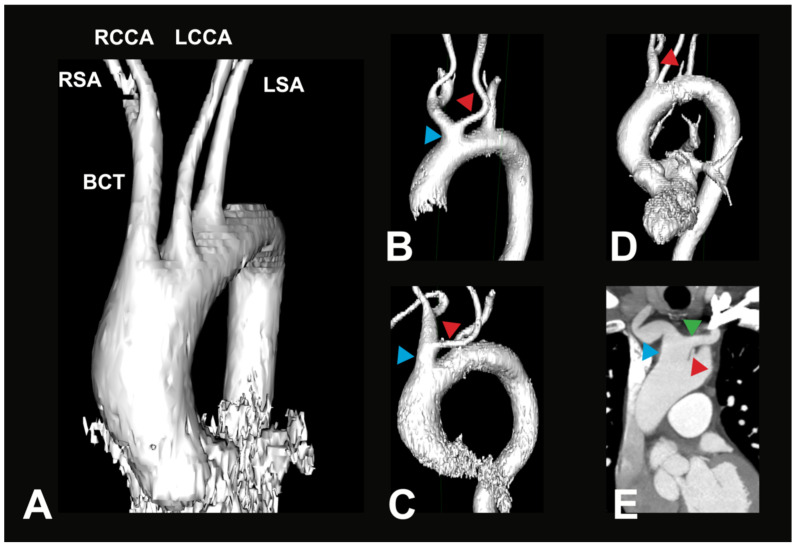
Most common AA variations. (A) Exemplary 3D reconstruction of a CTA in a 69-year-old female patient. Depicted is the most common variant of the branches of the aortic arch (V1). BCT—brachiocephalic trunk, RSA—right subclavian artery, RCCA—right common carotid artery, LSA—left subclavian artery, LCCA—left common carotid artery. (B) Exemplary 3D reconstruction of a CTA in a 66-year-old female patient. Depicted is a BCT variation (blue arrow), where the LCCA (red arrow) originates off the BCT in the vicinity of the aortic arch (V2a). (C) Exemplary 3D reconstruction of a CTA in a 90-year-old female patient. Depicted is a BCT variation (blue arrow), where the LCCA (red arrow) originates from a longer BCT (V2b). (D) Exemplary 3D reconstruction of a CTA in a 71-year-old male patient. Depicted is a direct origin of the left vertebral artery (LVA, red arrow) out of the aortic arch; all other arteries originate similarly as in the most common variant (V3a). (E) Paracoronal CTA in an 80-year-old male patient. Depicted is a direct origin of the left vertebral artery (LVA, red arrow) out of the aortic arch, in combination with a brachiocephalic trunk variation (blue arrow), where the LCCA (green arrow) originates from the BCT (V3b).
